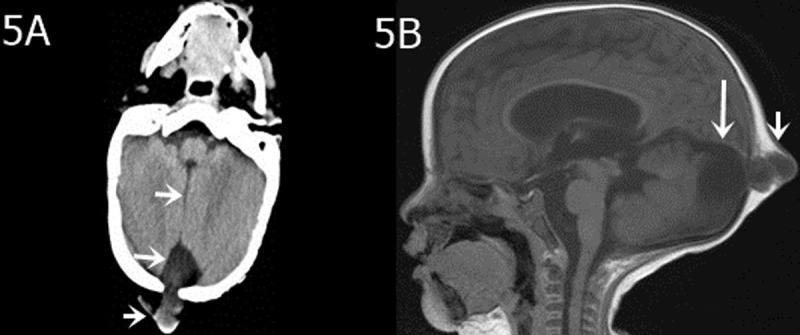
A seven-month-old girl with a pulsating occipital mass lasting seven months. A) The midline fissure (arrow) between the bilateral cerebellar hemispheres, the great occipital fossa (long arrow), and a localized occipital bone defect with accompanying meningeal and brain herniation (short arrow). B) Enlargement of the posterior fossa cistern (long arrow) and localized bony defect with accompanying meningeal herniation (short arrow) are visible in the sagittal T1-weighted image.
